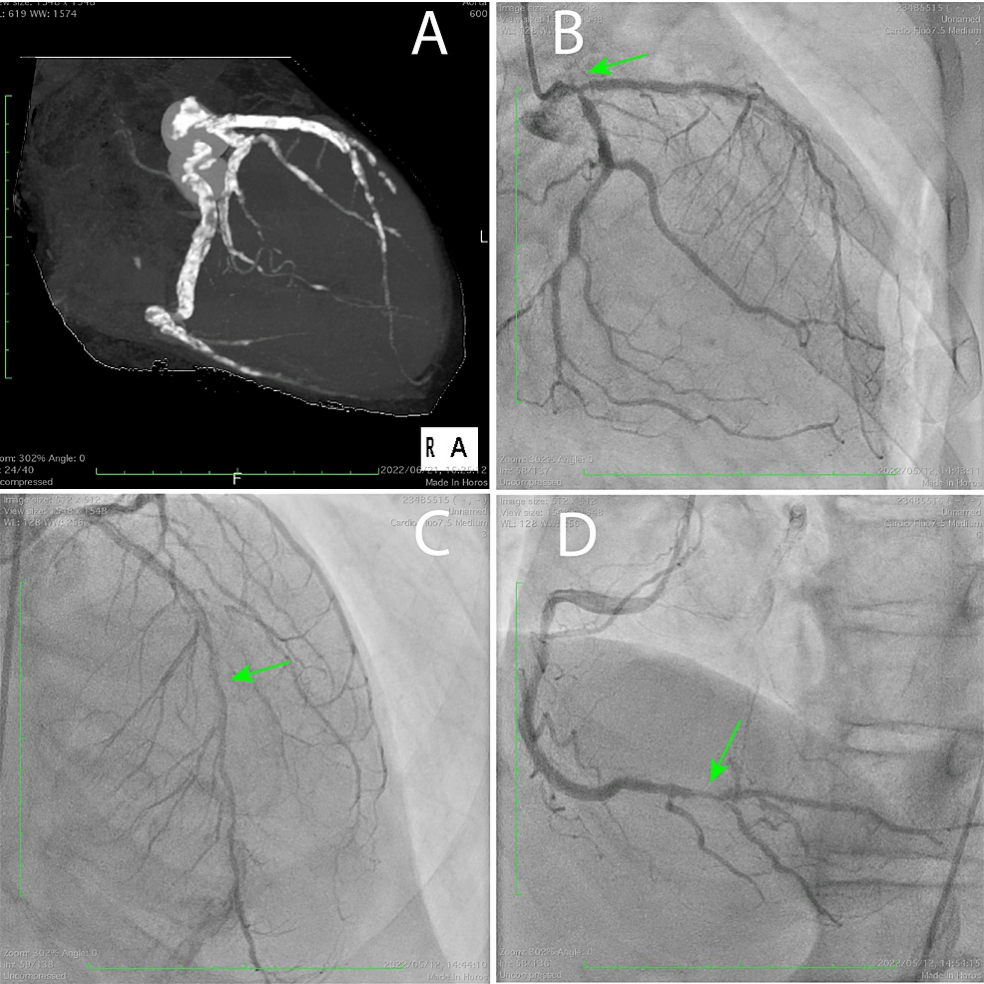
Preoperative findings. (A) Preoperative computed tomography scan maximum intensity projection. The patient exhibited triple-vessel coronary artery disease with severe calcification. (B) Left coronary artery angiography. The left main trunk had severe stenosis (green arrow). (C) Left coronary artery angiography. The left descending artery had severe stenosis (green arrow). (D) Right coronary artery angiography. Intermediate part had severe stenosis (green arrow).
